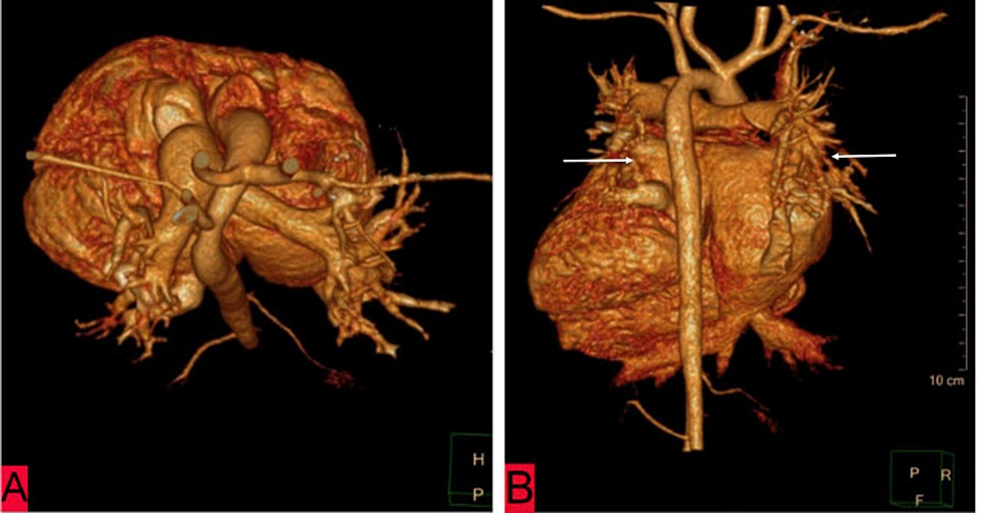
Reconstructed volume-rendered angiographic sections of chest CT of a five-year-old child suffering from heterotaxy syndrome revealing malposition of great vessels (A) and a veno-arterial discordance where the right-sided superior and inferior pulmonary veins were seen joining the right atrium, while the left-sided superior and inferior pulmonary veins were seen draining into the left atrium (B).
